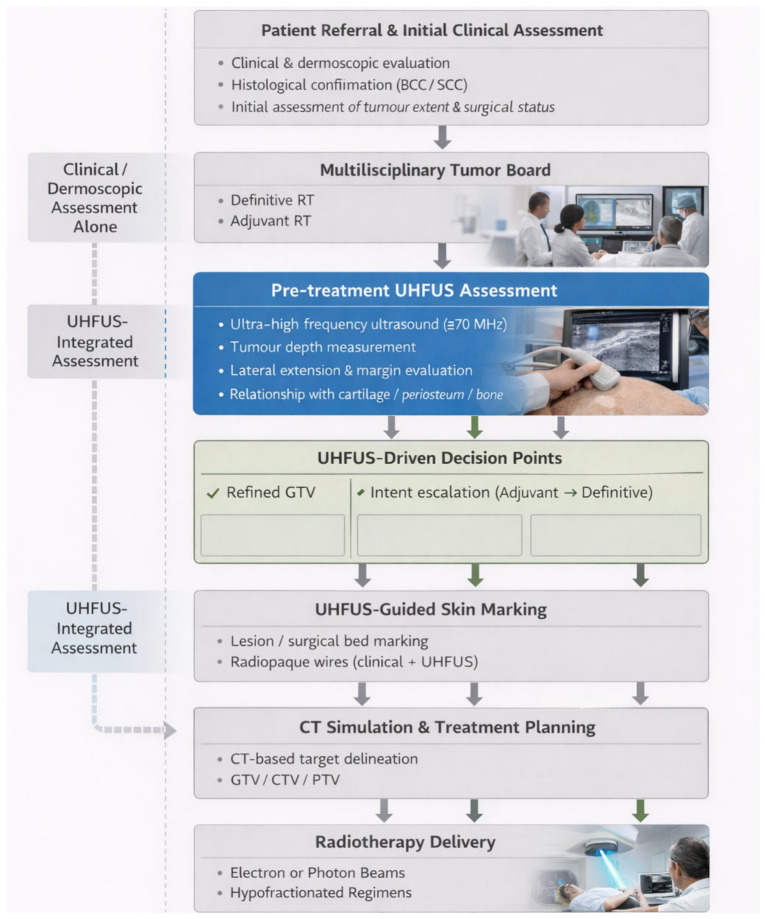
Structured ultra-high frequency ultrasound-integrated radiotherapy workflow.
